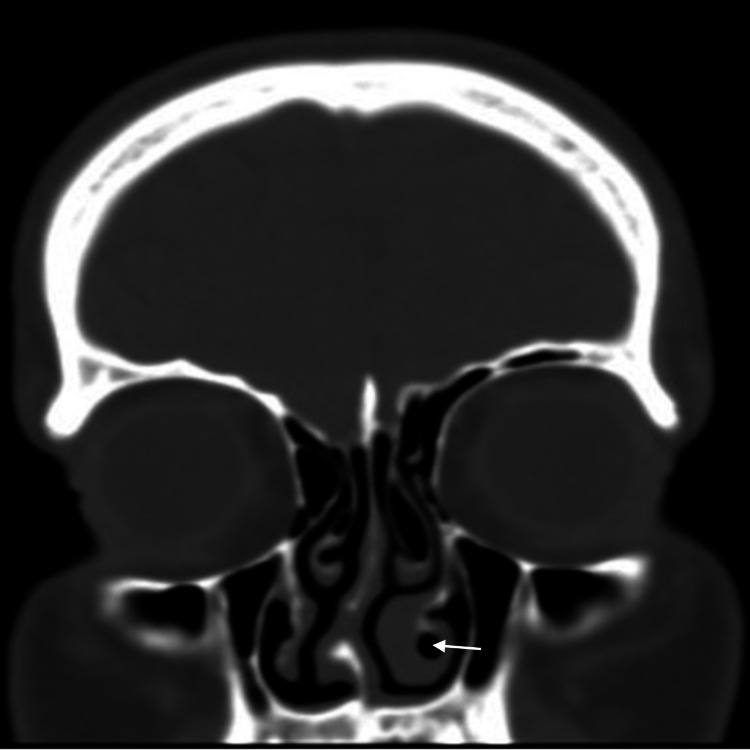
CT image of patient 1 (coronal view) Coronal view shows DNS to the right side, bilateral CB of the middle turbinates with right paradoxical middle turbinate, CB and hypertrophy of the left inferior turbinate (white arrow) DNS: Deviated Nasal Septum; CB: Concha Bullosa
